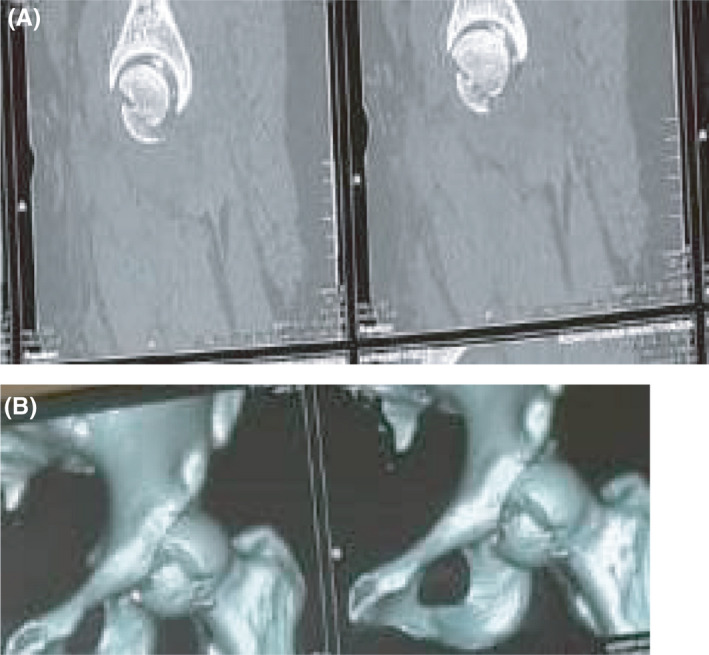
A and B, Postreduction axial CT scan (A) of the pelvis showing fracture of the femoral head with a nonanatomical reduction of the fragment and 3D reconstruction (B) showing femoral head fracture inferior to the fovea centralis (Pipkin I)
